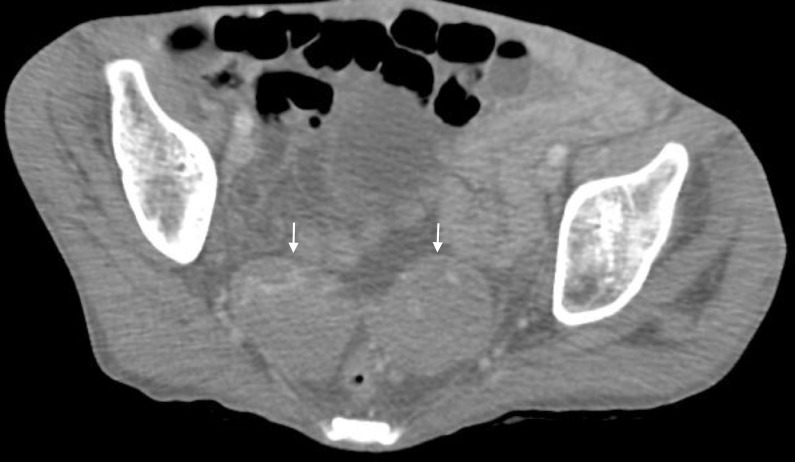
The sagittal cross section from the computed tomography (CT) of the pelvis of the bilateral adnexal masses.
